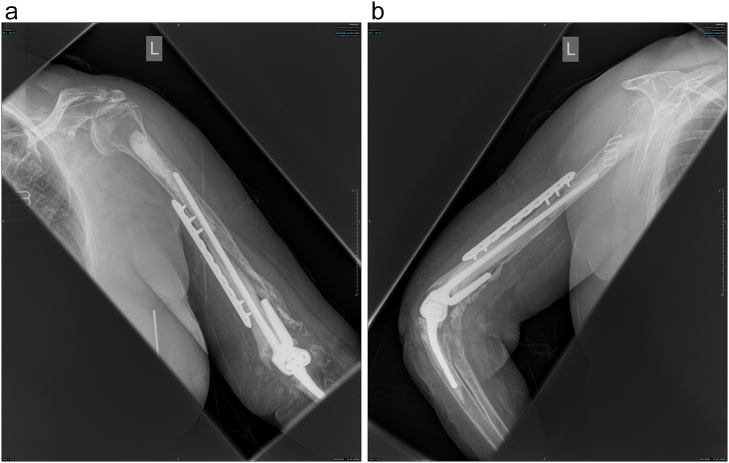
Postoperative radiographs, at 6 months, demonstrating the intact humeral allograft-plate-construct, no evidence of loosening of the humeral stem, and osseous union at the step-cut allograft humeral-host interface.
